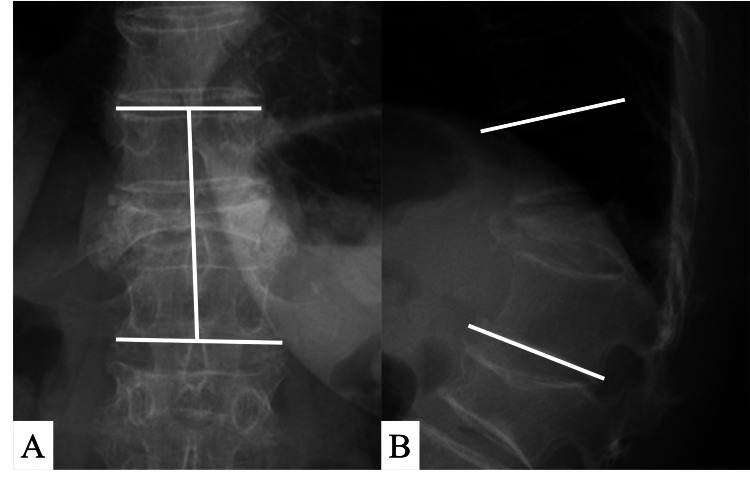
Radiologic parameters. A: The coronal Cobb angle was measured at the angle between the intact endplates cranial and caudal to the fractured vertebra on an anterior-posterior radiograph. The intervertebral height was measured between the anterior mar­gins of the intact endplates cranial and caudal to the fractured vertebra. B: The kyphotic angle on a lateral radiograph was measured by calculat­ing the angle of the intact endplates cranial and caudal to the frac­tured vertebra to evaluate the mean sagittal correction.
